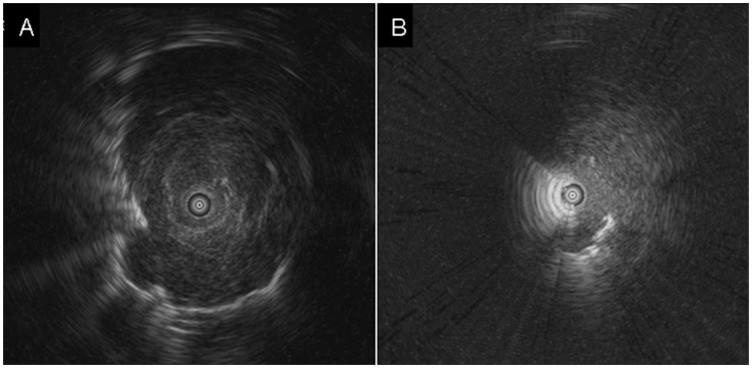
Endobronchial ultrasound images showing that the probe was located (A) within the lesion and (B) adjacent to the lesion.
